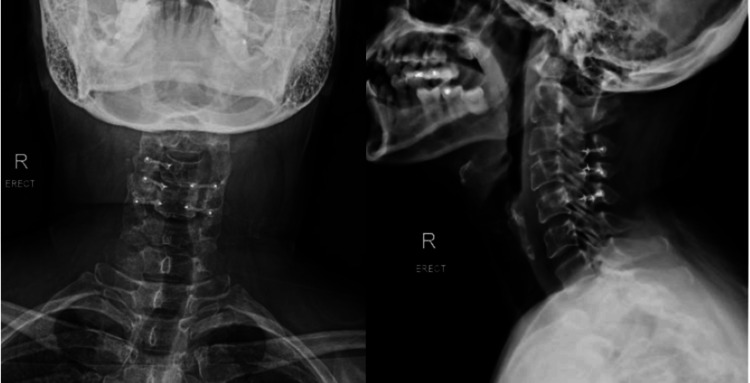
Post-operative X-ray images demonstrating satisfactory placement of Synthes cranial plates and screws.
